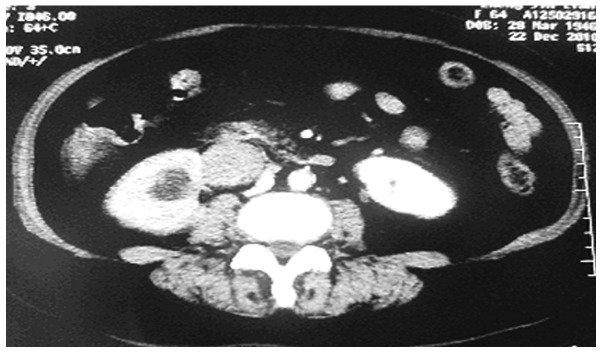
CT scan revealing a mass measuring ~5×4 cm in the right ureter, with light hydronephrosis. CT, computed tomography.
